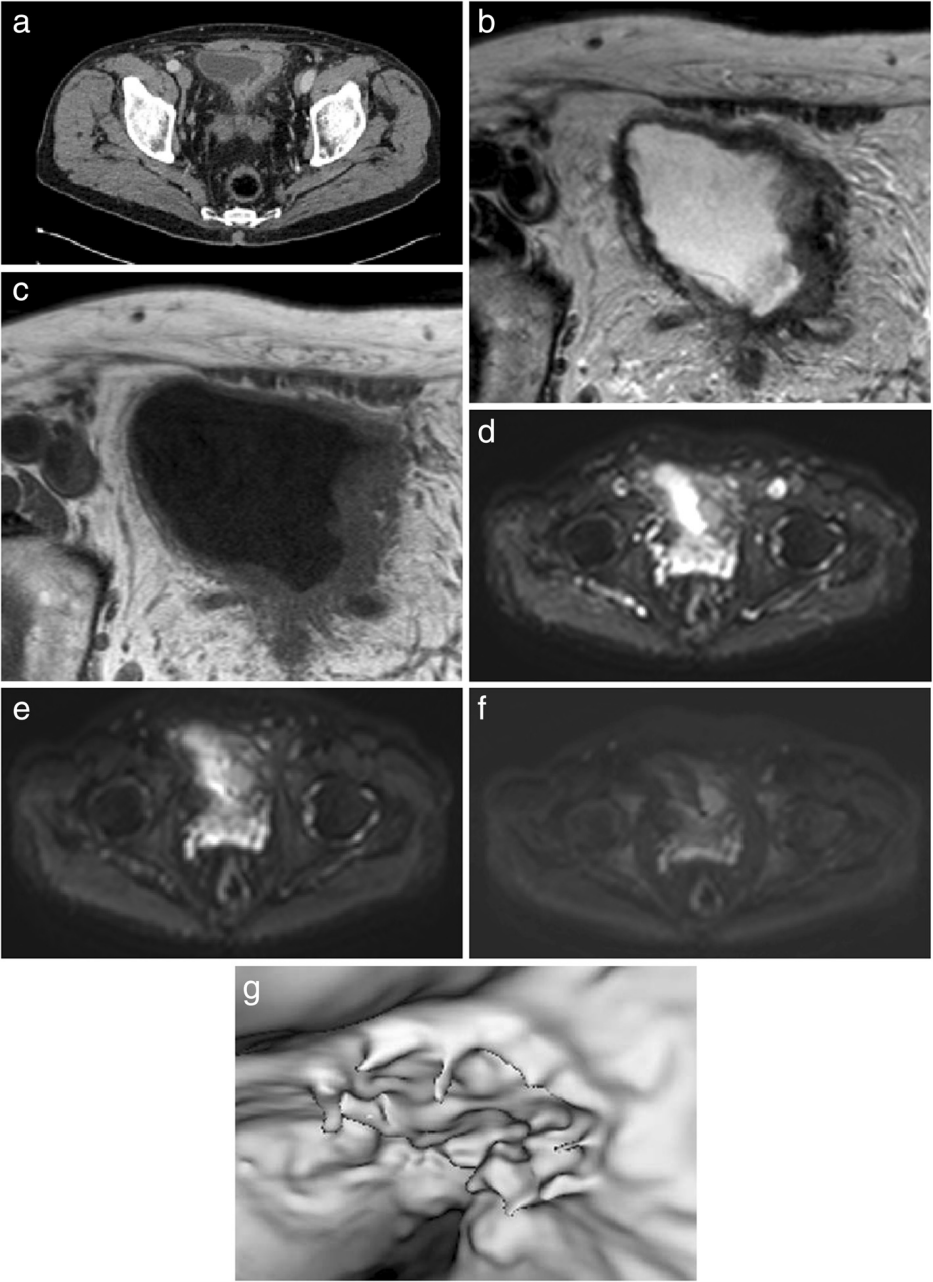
Patient with known T2 N0 M0 bladder cancer (left bladder wall): (a) contrast-enhanced computed tomography (CT) scan, (b) axial T2-weighted image performed on a 3 T magnetic resonance imaging (MRI) unit showing a hypointense lesion, (c) corresponding T1 image, (d) axial diffusion-weighted (DW) MRI at b-value 0, (e) axial DW MRI at b-value 100, (f) axial DW MRI at b-value 750, and (g) magnetic resonance virtual cystoscopy (MRVC) of same tumor with three-dimensional reconstruction of tumor bed showing opening into adjacent diverticulum.
